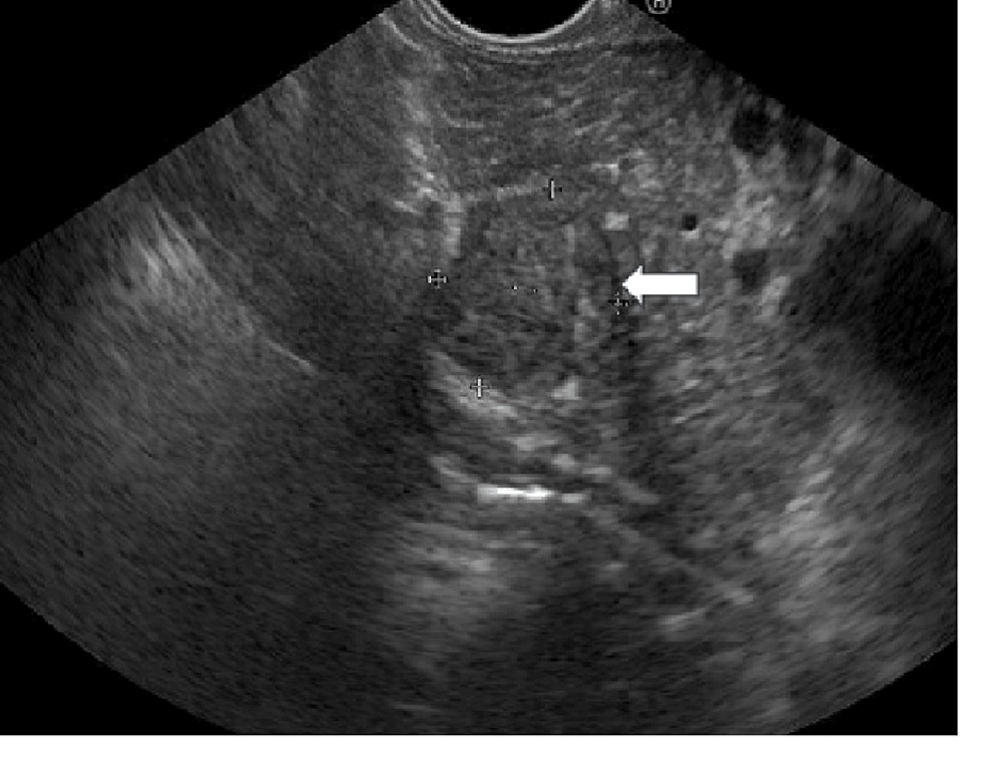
Endoscopic ultrasound showing a hypoechoic mass lesion of 19x18 mm (arrow) between the pancreatic neck and gastric wall
